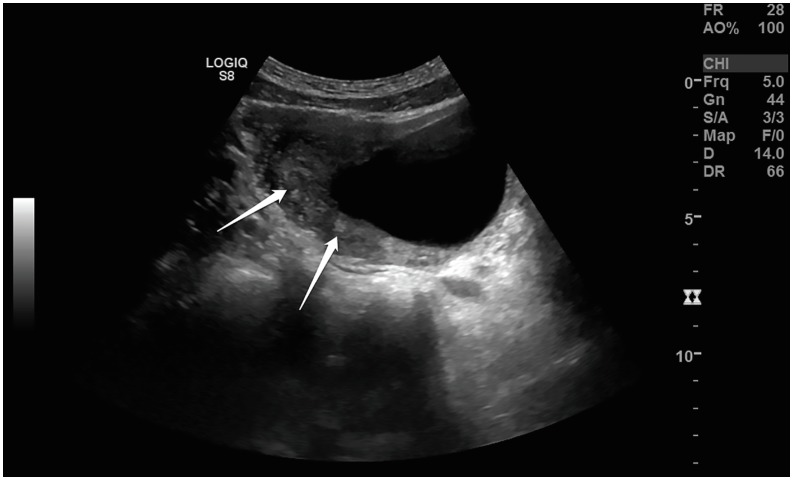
Ultrasound imaging; Diffuse thickening of the anterosuperior and posterior walls of the bladder and multiple round, <5mm nodular echogenities in the thickened walls marked with arrows.
